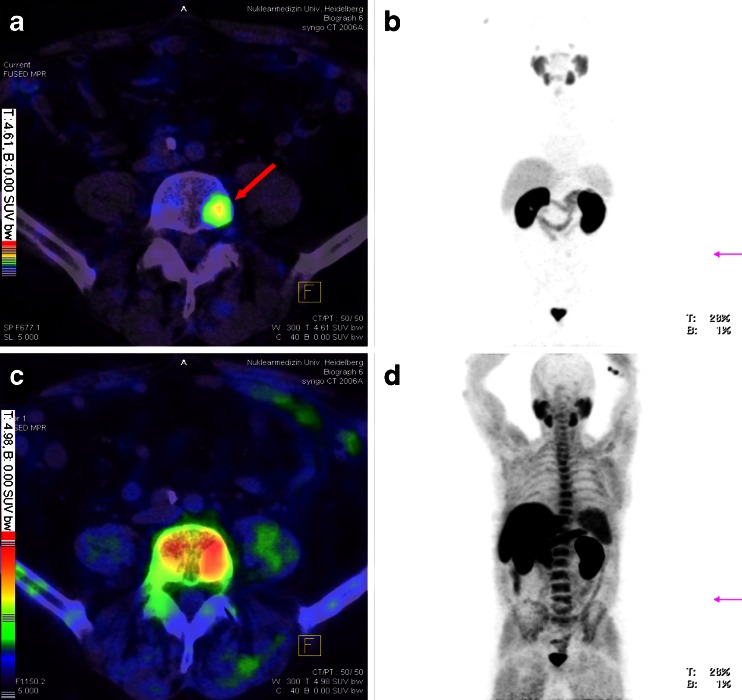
Patient 15. *Red arrow* points to a vertebral metastasis visible in ^68^Ga-PSMA PET/CT (a) only. Due to physiological high background activity in the vertebral column, vertebral metastases are usually difficult to detect in choline PET (c). Typical for choline PET is also a frequently high background activity as visible in the maximum intensity projection (MIP, d). Image data are given as automatically produced by the PET/CT machine to demonstrate that the filtering of the MIPs was equally set. Although it appears that the automatically set scales may not be optimal to detect lesions on the MIP in every case (e.g. lesion in b is better visualized on the workstation than in this figure), we preferred to avoid changes of all figures presented in this study. a Fusion of ^68^Ga-PSMA PET and CT, b MIP of ^68^Ga-PSMA PET, c fusion of ^18^F-fluoromethylcholine PET and CT, d MIP of ^18^F-fluoromethylcholine PET. Colour scales as automatically produced by the PET/CT machine
